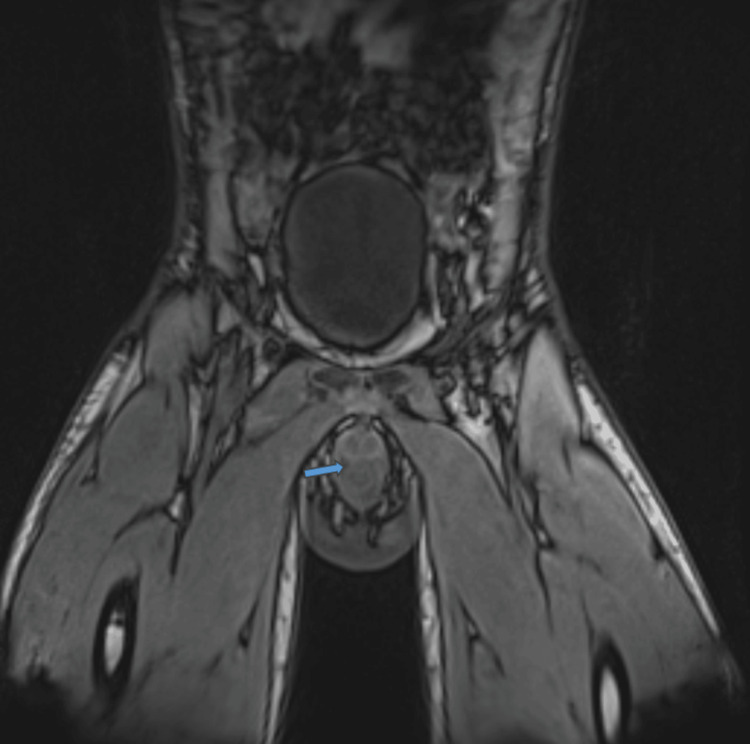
Pelvic MRI: a small tear involving the right lateral aspect of the bulbar urethra, measuring approximately 2.5 mm, along with a peri-urethral collection.
